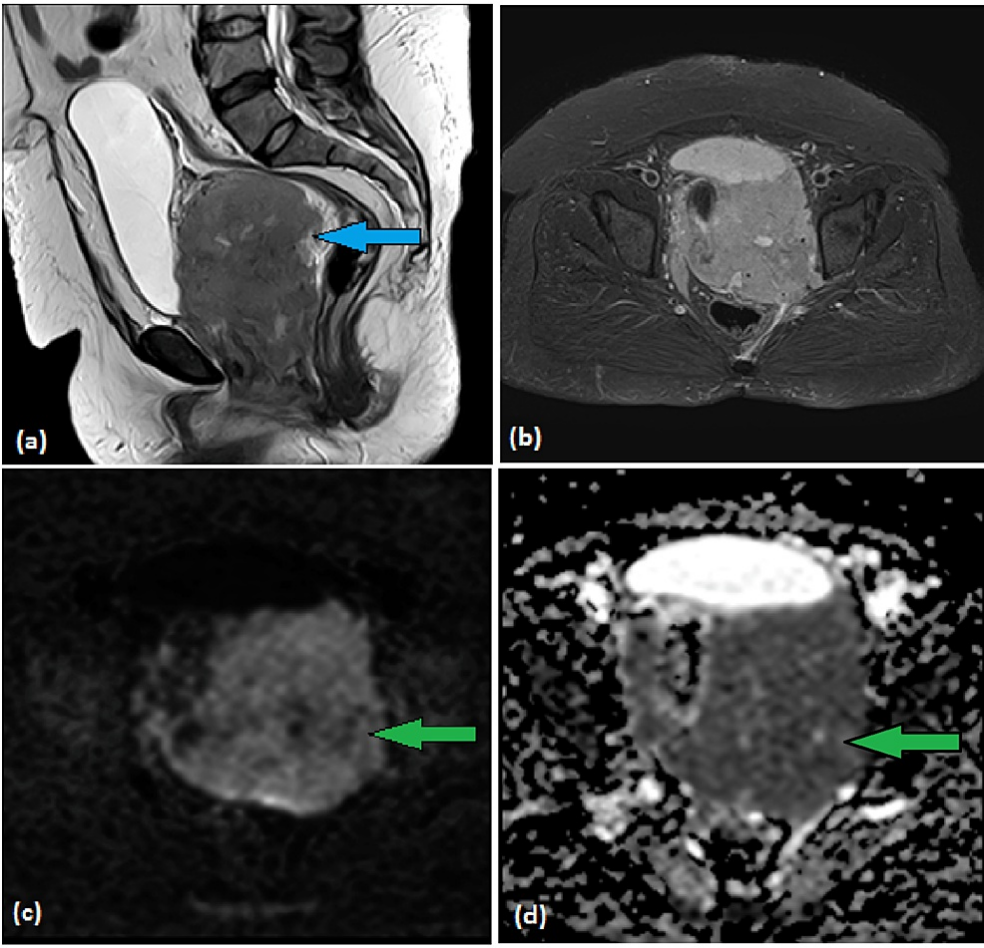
MRI T2W sagittal (a), axial STIR (b), DWI (c), and ADC (d) images demonstrating large vaginal mass showing low signals on T2W (blue arrow) and high signals with diffusion restriction (green arrows). T2W: T2 weighted, STIR: Short tau inversion recovery, DWI: Diffusion-weighted imaging, ADC: Apparent diffusion coefficient
